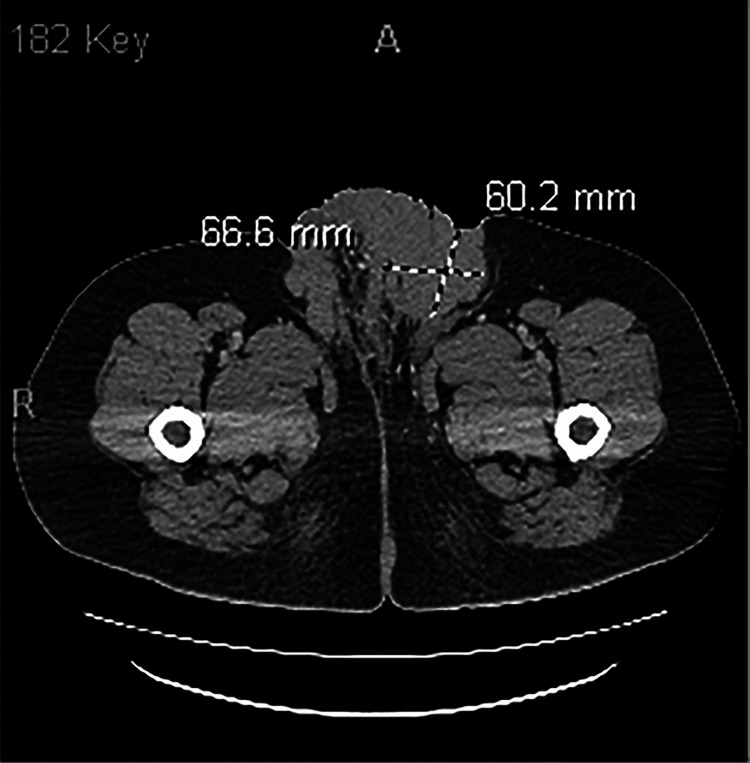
2025 CT abdomen and pelvis w/ intravenous (IV) contrast only (multiple volumetric CT images of the abdomen and pelvis were obtained from lung bases to pubic symphysis with IV contrast for evaluation for necrotizing fasciitis/hx of giant condyloma). Interval enlargement of coalescent fungating lesions of the bilateral groin, scrotum, and perineum with central gas foci (necrosis vs. skin folds), concerning for infiltrative neoplasm; superimposed infection not excluded. Minimal fluid along the left anteromedial thigh fascia without fascial gas. Extensive pelvic and inguinal lymphadenopathy.
